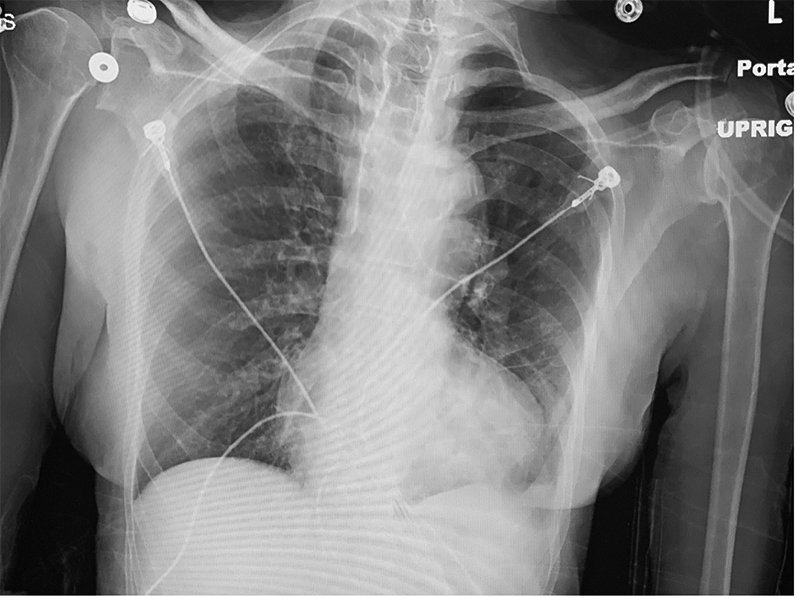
Chest X-Ray Film No acute pulmonary pathology.
